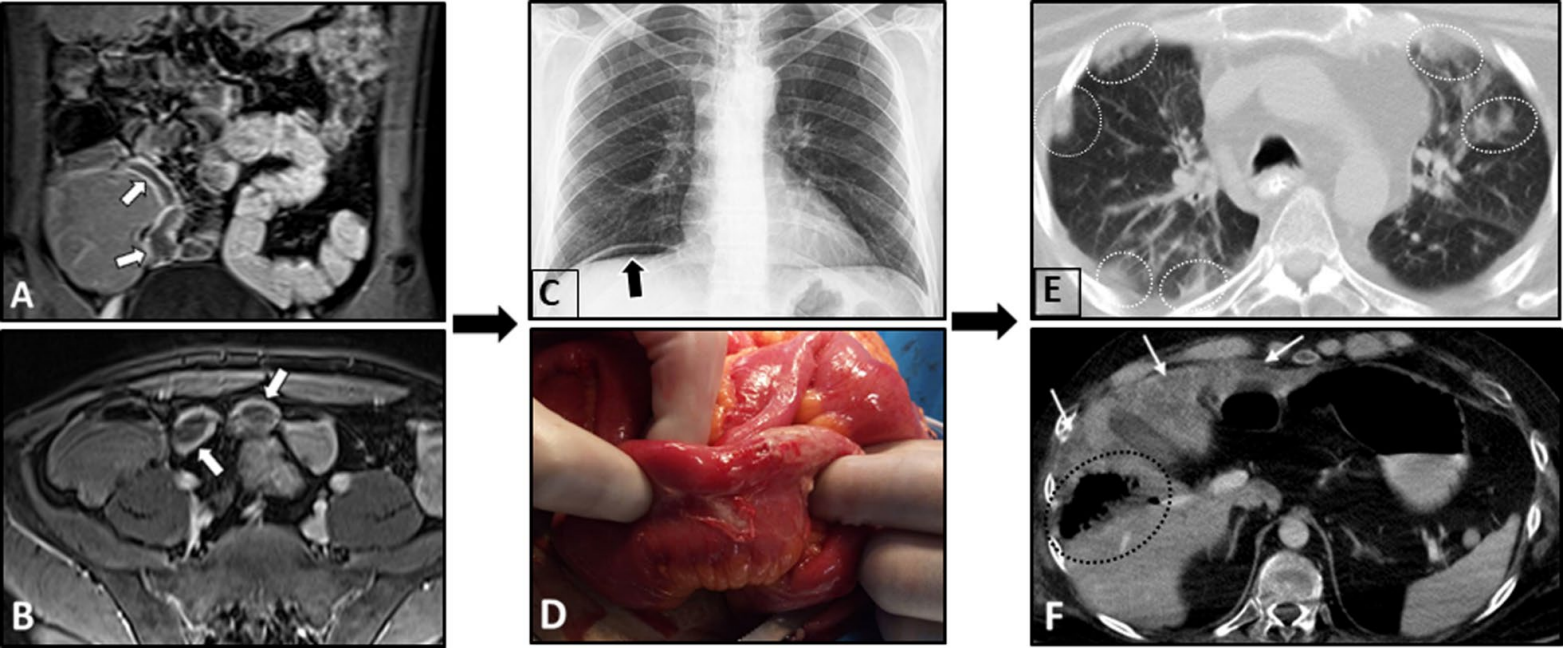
Intestinal vasculitis and perforation in a 32-year-old male with a history of asthma presenting with abdominal pain and eosinophilia. MRE was obtained. Coronal and axial post-contrast T1-W images (A, B) display increased mural enhancement at the terminal ileum without thickening (thick white arrows). Three months later (C, D), the patient presents to the ER with acute abdominal pain without definite diagnosis or treatment. Chest X-ray (C) shows subdiaphragmatic free gas (thick black arrow). He underwent an emergency laparotomy. Intraoperative photograph (D) revealed focal bowel perforation at the distal ileal segment. After segmental bowel resection, an ileostomy was performed. Eight months after surgery (E, F), he was admitted for RUQ pain and dyspnea. Axial chest CT image (E) demonstrates bilateral GGO and consolidations with lobular distribution (white dotted oval). Axial contrast-enhanced CT image (F) shows multiple wedge-shaped areas of hypoattenuation in the liver (thin white arrows), consistent with hepatic infarction. Intraparenchymal gas formation is also evident at the infarcted segment, suggestive for abscess formation (black dotted oval). Small-vessel vasculitis was confirmed based on clinical/laboratory data and pathology results suggestive of Churg–Strauss syndrome. Unfortunately, he died secondary to sepsis
